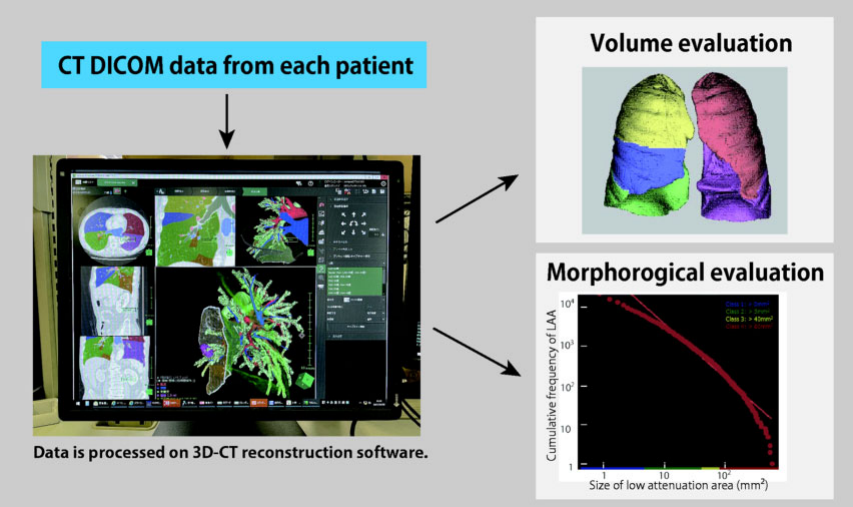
Volumetric and morphological analyses on the 3D CT software. 3D CT: 3-dimensional computed tomography; DICOM: Digital Imaging and Communications in Medicine; LAA: low-attenuation area.
